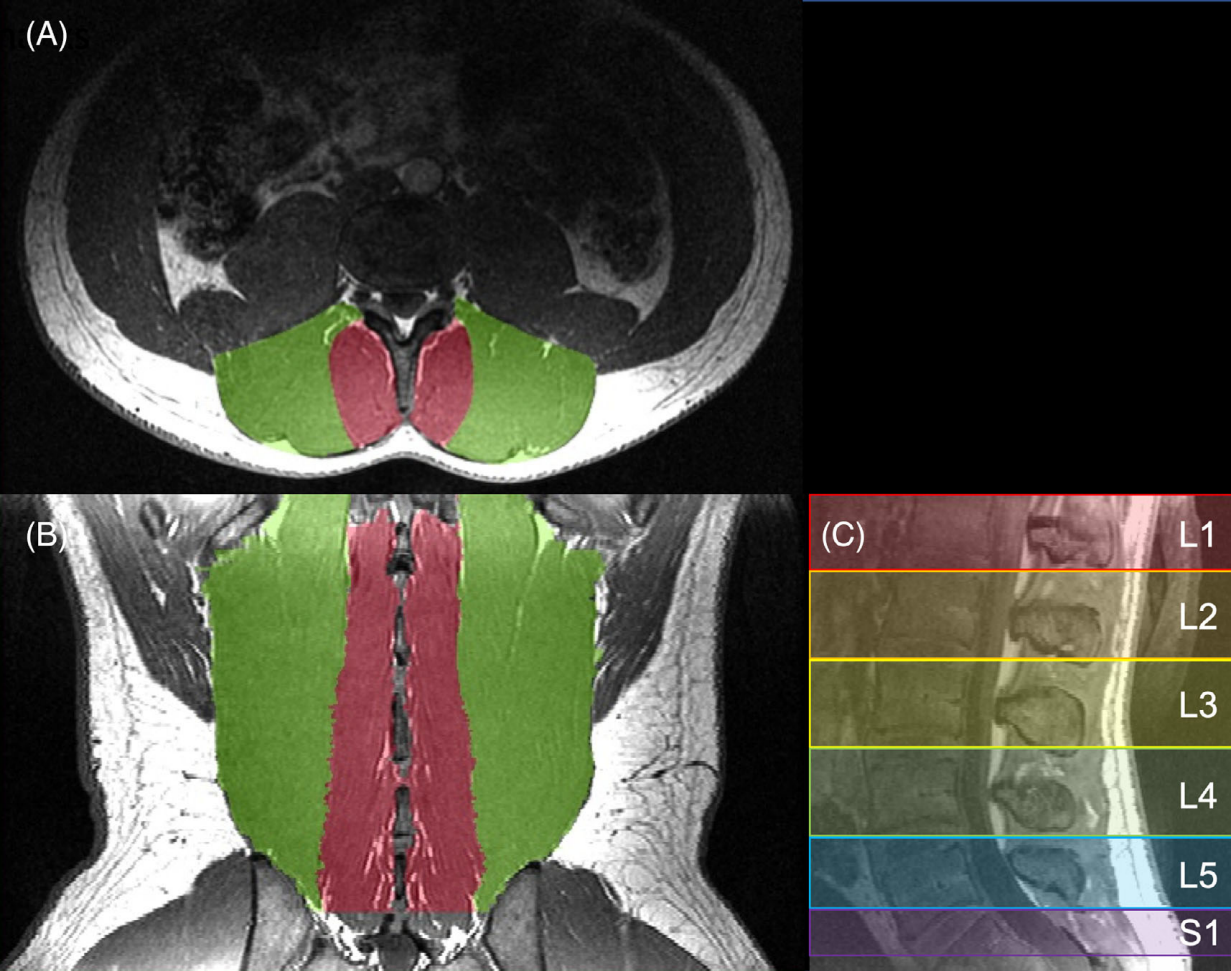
Sample anatomical MRIs used for segmentation of the multifidus (red) and erector spinae (green). A, Segmentations were manually drawn in OsiriX in the axial plane. B, Coronal reformatted MRI of the multifidus and erector spinae muscle masks. C, Mid‐sagittal reformatted MRI demonstrating how level dependent measures were segmented from the midpoint between adjacent vertebrae
